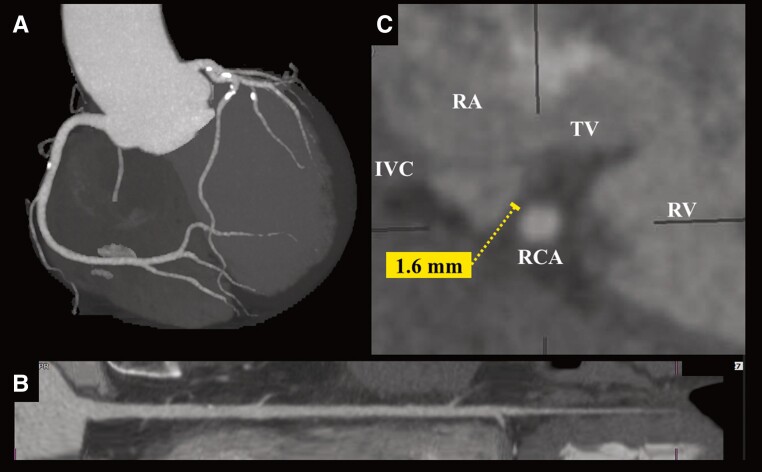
3D coronary computed tomography imaging shown in the left anterior oblique view (*A*), straight view of the right coronary artery (*B*), and trans-axis cross-sectional view at the level of the cavo-tricuspid isthmus (CTI) ablation line (*C*) before the cavo-tricuspid isthmus ablation. IVC, inferior vena cava; RA, right atrium; RCA, right coronary artery; RV, right ventricle; TV, tricuspid valve.
